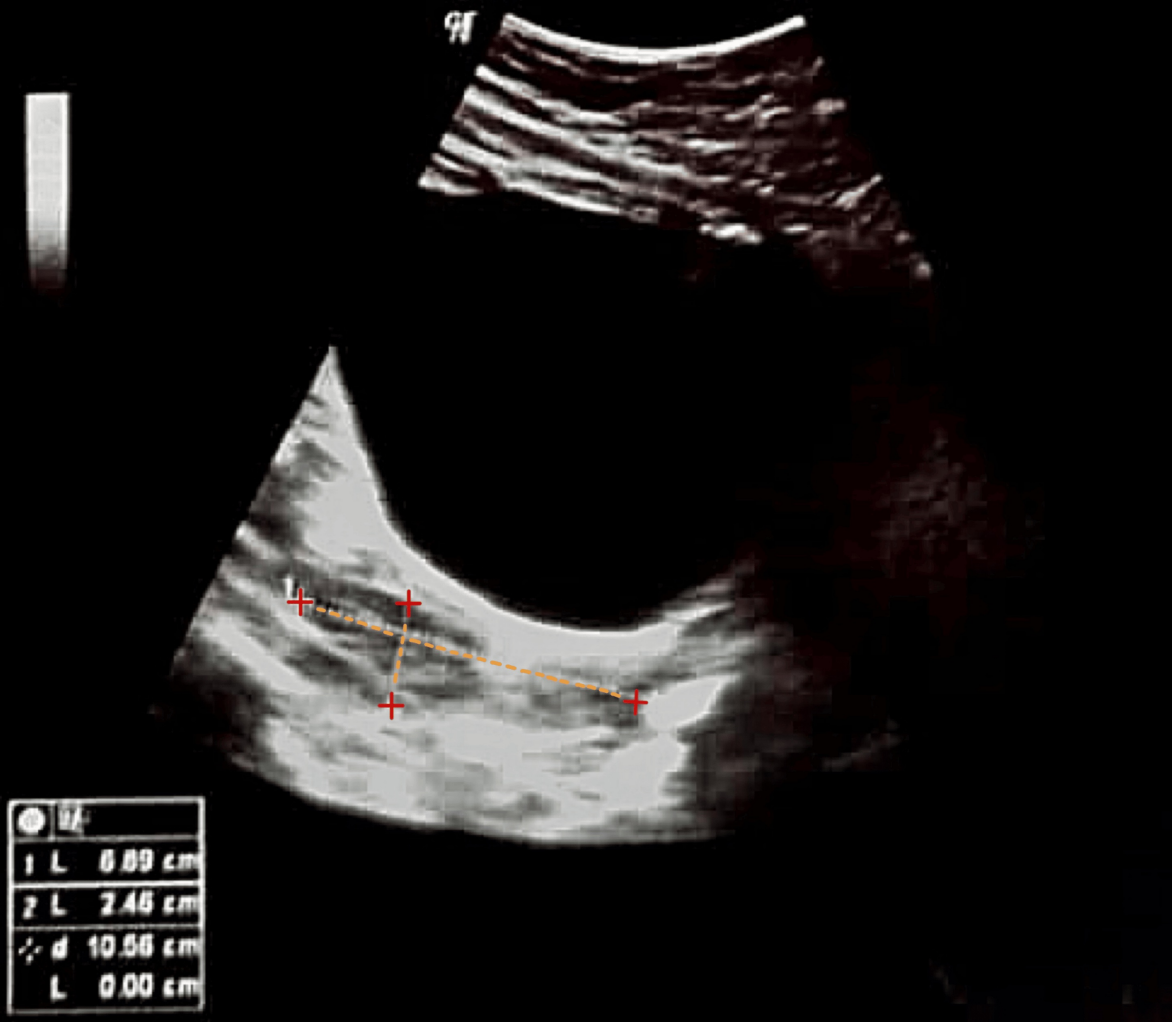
Transvaginal ultrasound of the endometrial lesion showing increased endometrial thickness.
